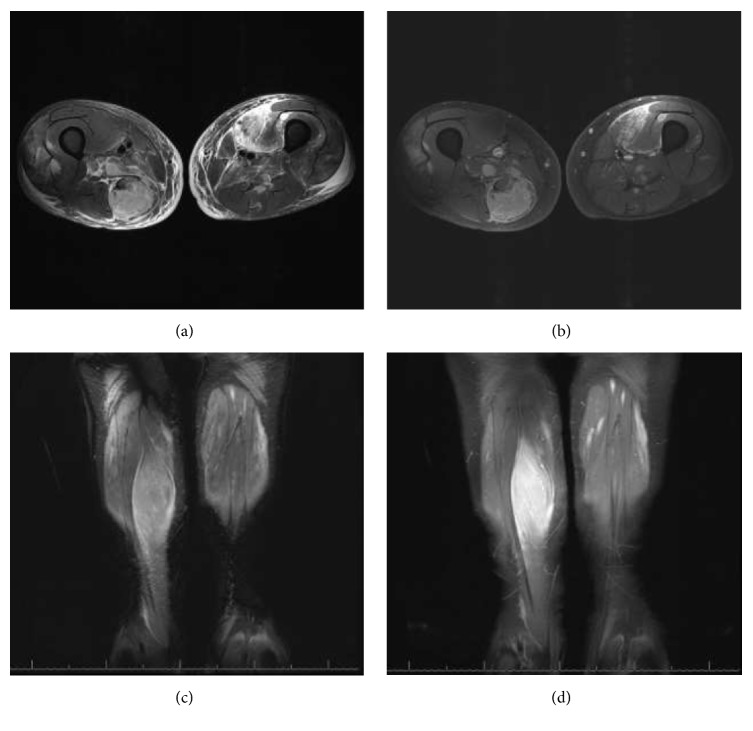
Lower extremity MRI for the same 51-year-old patient obtained a few days after the initial US. (a) is an axial T2 weighted fat suppressed image and (c) is a coronal T2 weighted fat suppressed image of the thighs. The images show multiple T2 hyperintense lesions. The largest lesion on the distal right thigh corresponds to the heterogeneous lesion seen on a week-earlier US. (b, d) are axial and coronal T1 fat suppressed postcontrast images, demonstrating associated enhancement of those lesions. Given the patient history of multiple myeloma, those lesions were suspected to represent extra-axial multiple myeloma, which was confirmed with surgical biopsy few days later.
